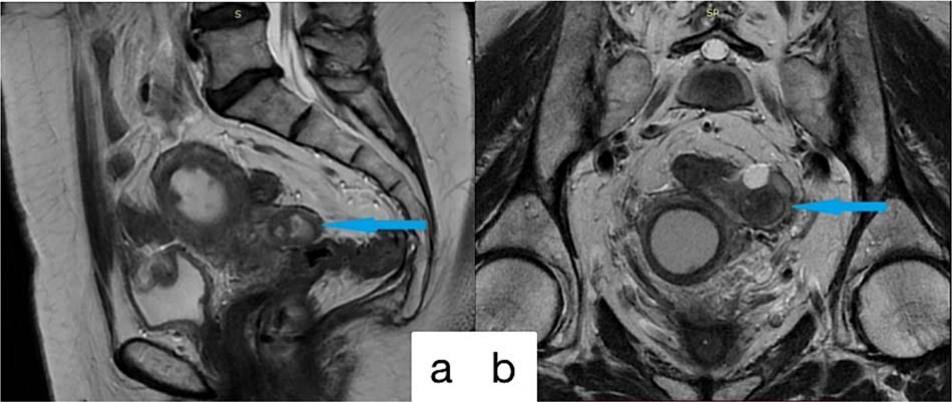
Preoperative MRI imagines; (a) sagittal; (b) coronal; alterations in the left fallopian tube, enlargement of the uterine cavity and cervical canal, and soft tissue hyperplasia within the uterine cavity.
